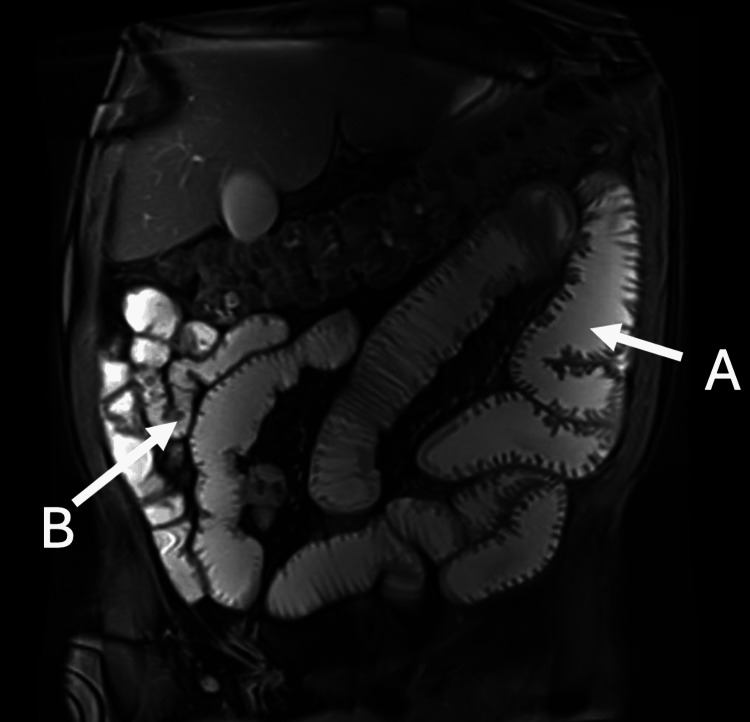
T2-weighted coronal view of MRI enterography. (A) Dilated proximal small bowel loops (B) apparent transition point with collapsed distal small bowel near the terminal ileum.
